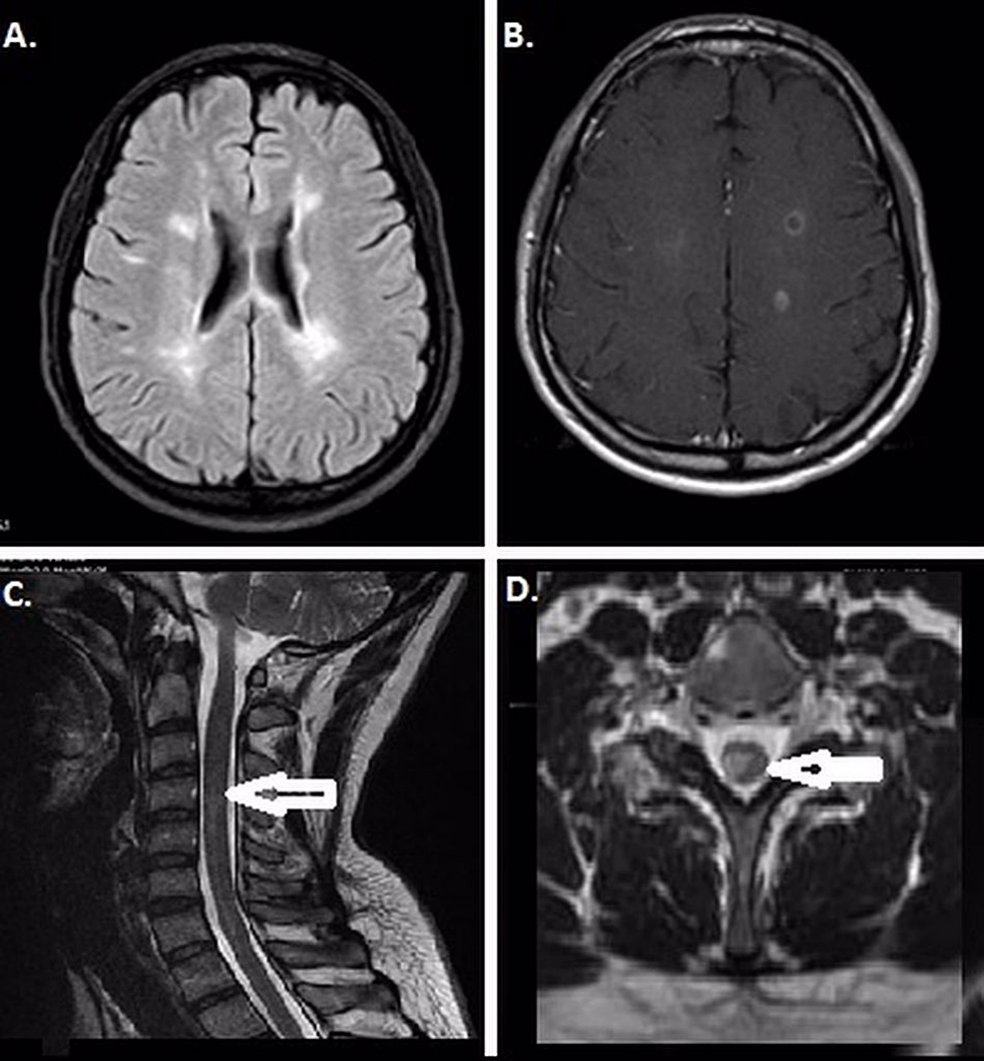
Case 3 Hyperintense lesions in the periventricular area in an axial FLAIR (A), and contrast enhancement lesions involving centrum semiovale in axial T1 sequences (B). After two months, note the new contrast-enhancing lesions in the cervical spinal cord in sagittal (C) and axial T2 sequences (D).
